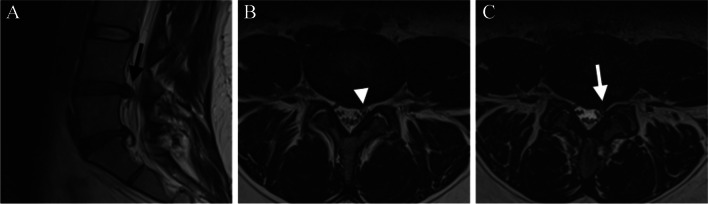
Evaluation of lumbar recess stenosis on 2D and 3D MRI sequences. A typical L4/5 disc herniation is seen on conventional 2D T2-weighted sagittal MR image (A). In the routine 2D T2 TSE axial image no recess stenosis is visible (white arrowhead) (B), whereas in the 3D T2 TSE (SPACE) image a definite stenosis is seen in the left lateral recess (white arrow) (C)
